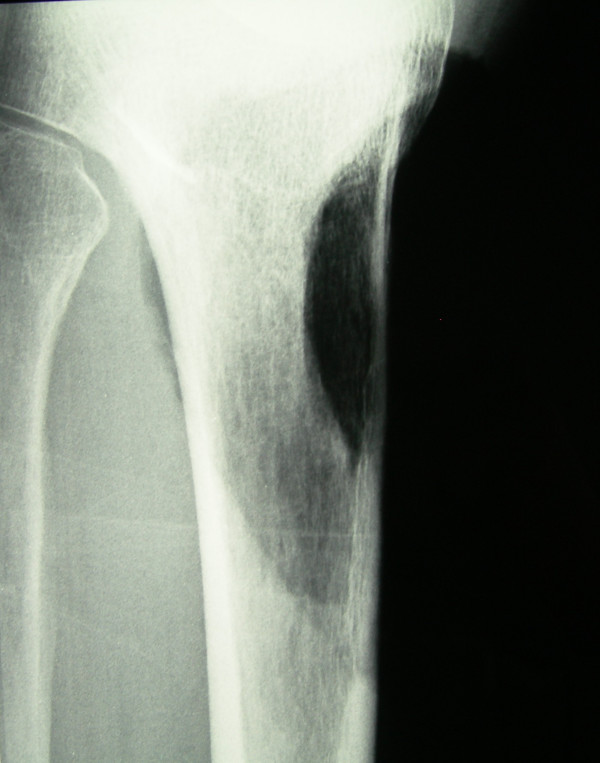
Post-operative Anteroposterior X-Ray view of the proximal tibia. Bone resection was decided for the achievement of wide and tumor-free surgical margins.
